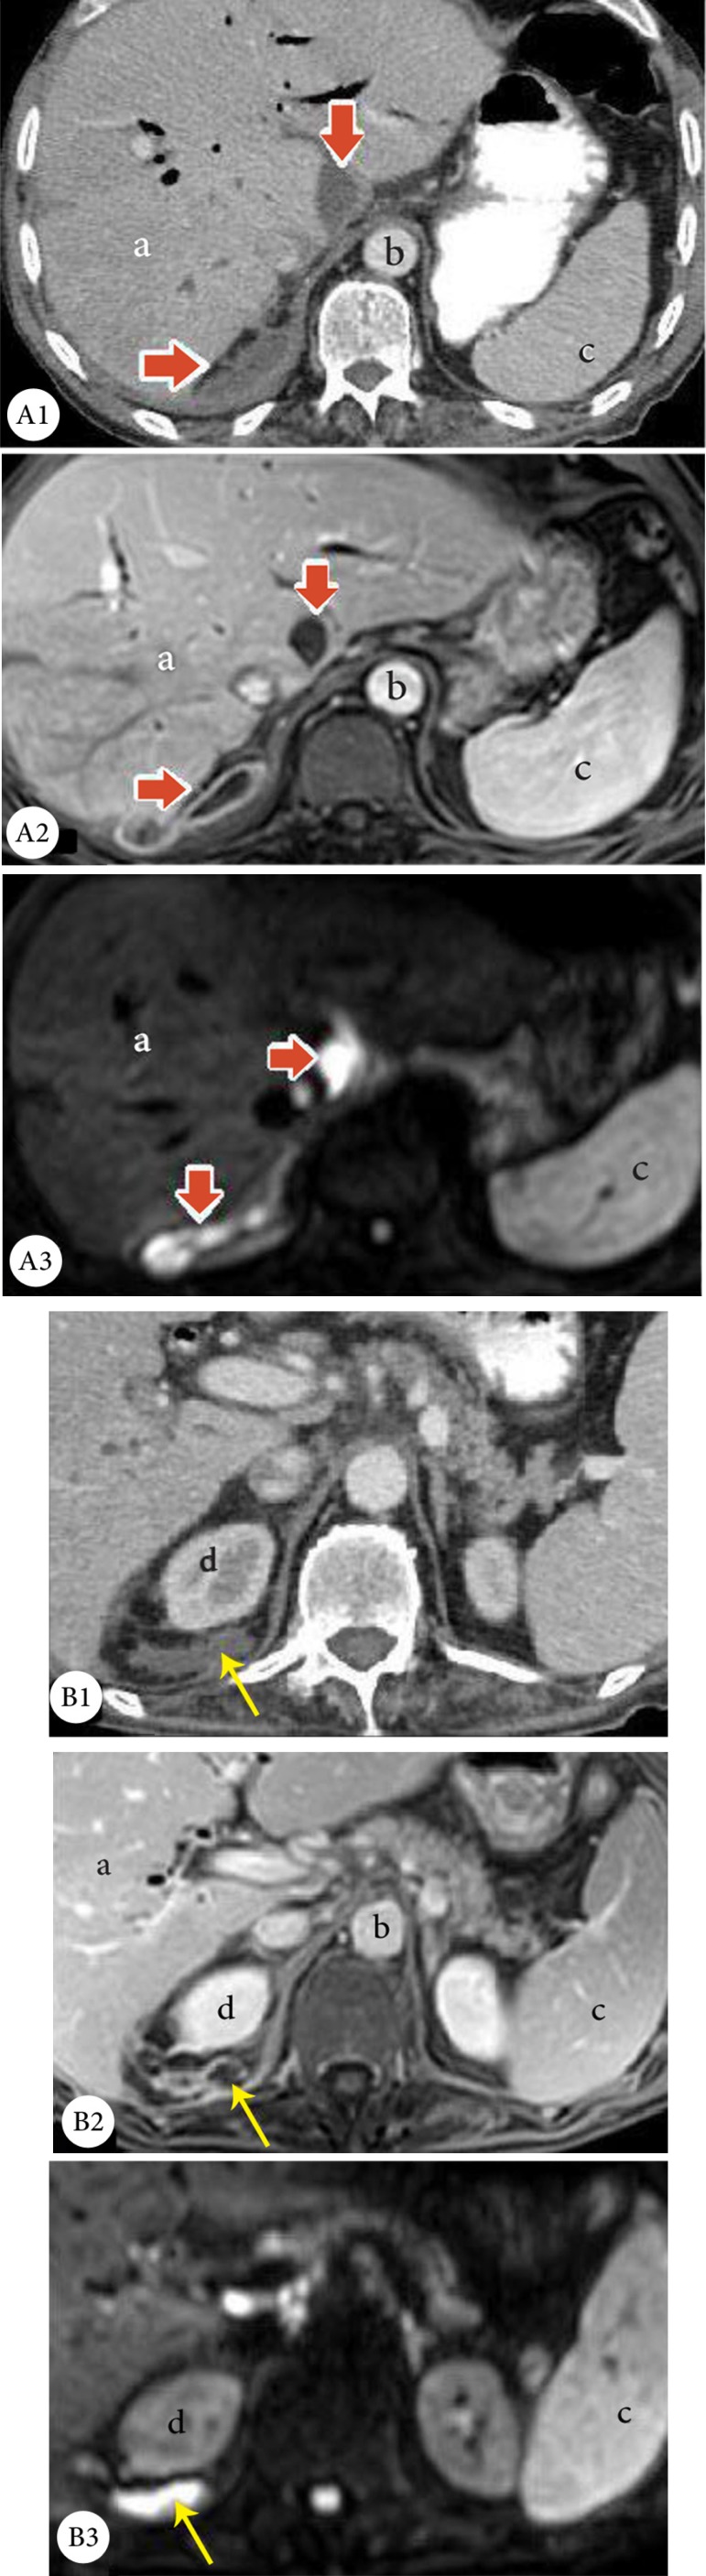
Abdominal contrast-enhanced computerized tomography axial (CT) scan, showing a ring-enhanced collection in the retrohepatic space, medial to the caudate lobe arrows (Panel A1) and the right perinephric space (arrow in Panel B1). Abdominal contrast-enhanced T1 magnetic resonance imaging (MRI), showing the non-central enhancing collection with rim enhancement in the contrast-enhanced T1-spoiled gradient echo image (arrows in Panels A2 and B2). Abdominal diffusion weighted MRI, showing a high signal collection (b-value=1000), in favor of abscess formation (Panels A3 and B3).
